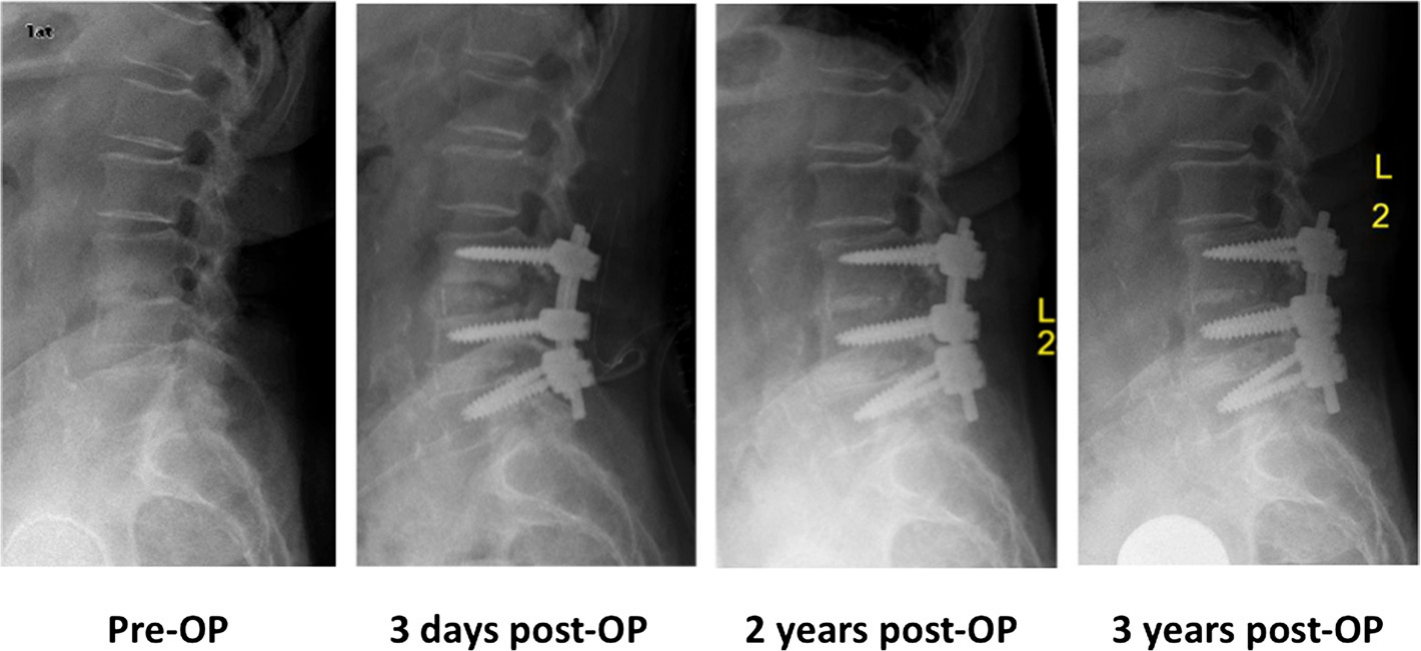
Pre- and postoperative conventional X-rays in the lateral view (*upright standing*) throughout the 3 year follow-up. In addition, the patient received a total hip arthroplasty of the left hip due to progressive hip arthritis, 2 years after her uneventful recovery from the lumbar spine surgery
